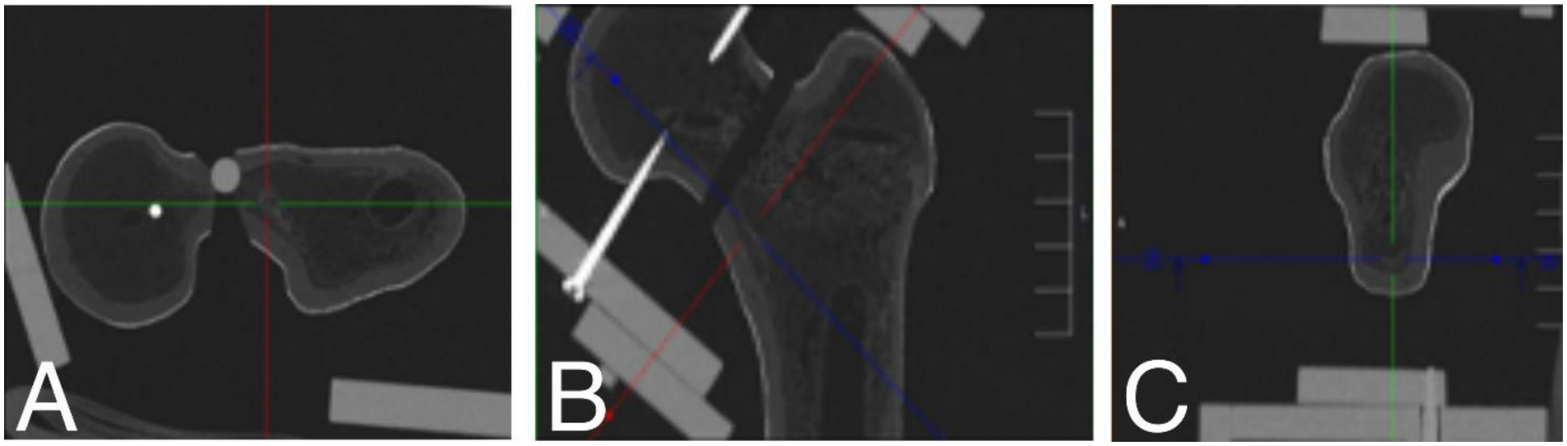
An exemplary 3D CT scan of the synthetic femoral bone model fixed by means of the goniometer is shown. The alignment of section planes in all three dimensions is depicted: A axial, B coronal and C sagittal view using Visage^*®*^.
